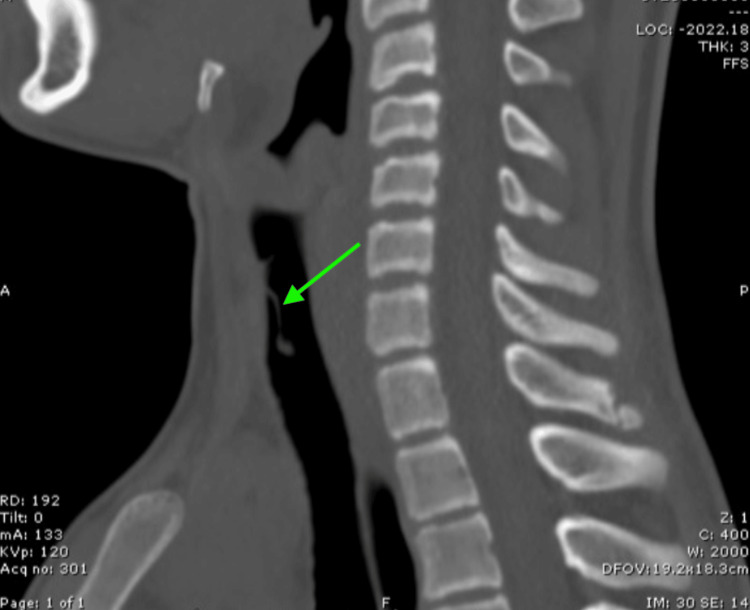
Noncontrast bone-window CT scan with arrow pointing to the foreign body in the subglottic airway.
